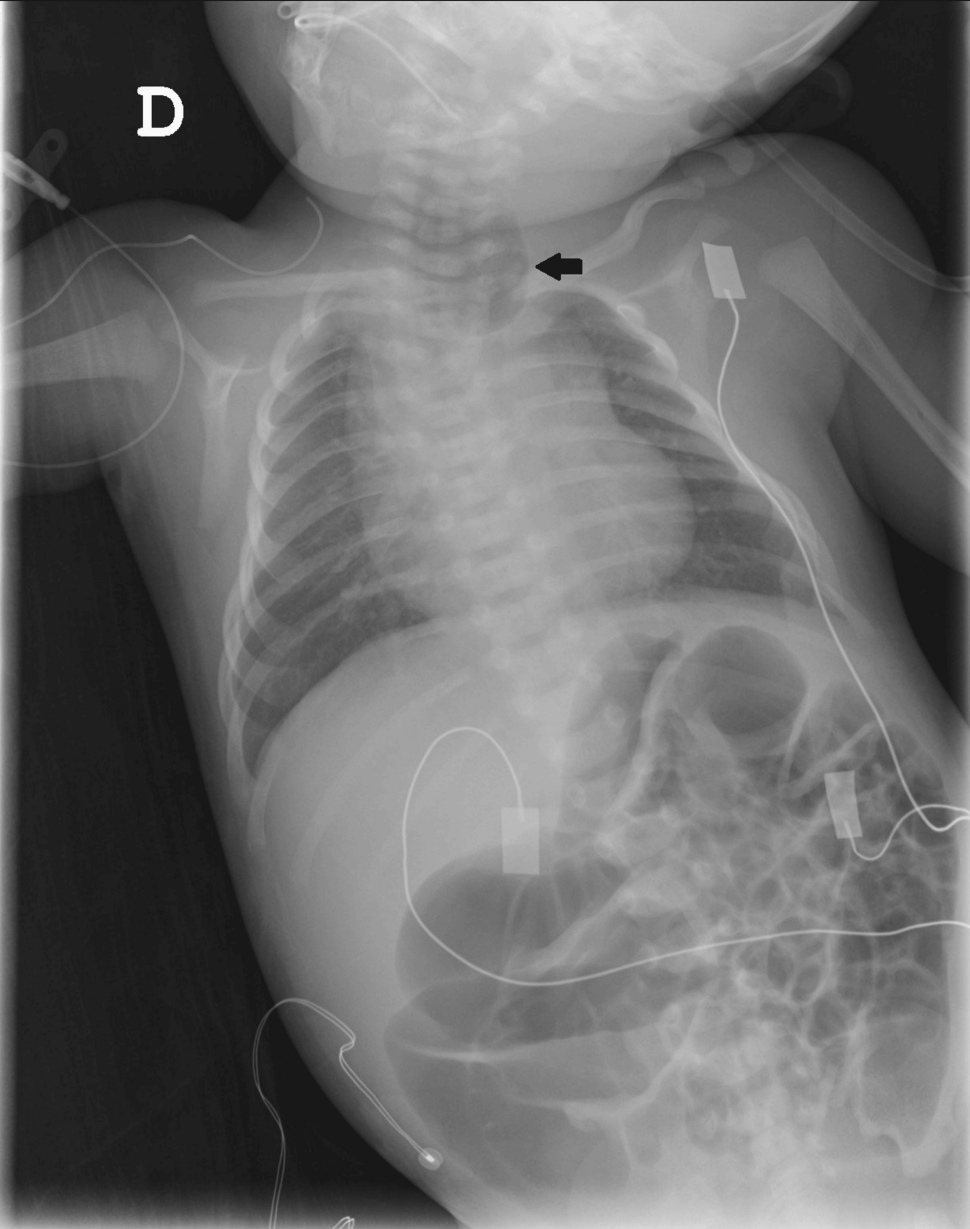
Thoracic radiograph Frontal chest radiograph demonstrating an esophageal pouch (black arrow) and air in the stomach and intestine, compatible with esophageal atresia with distal fistula.
